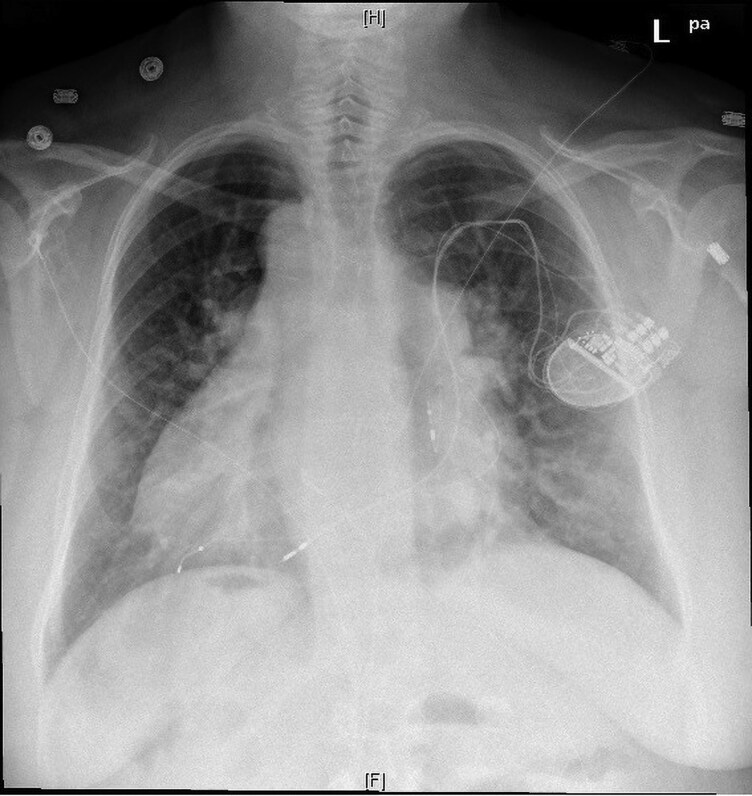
PA chest x-ray demonstrating CRT-P implant with bipolar LV lead and lead positions.
